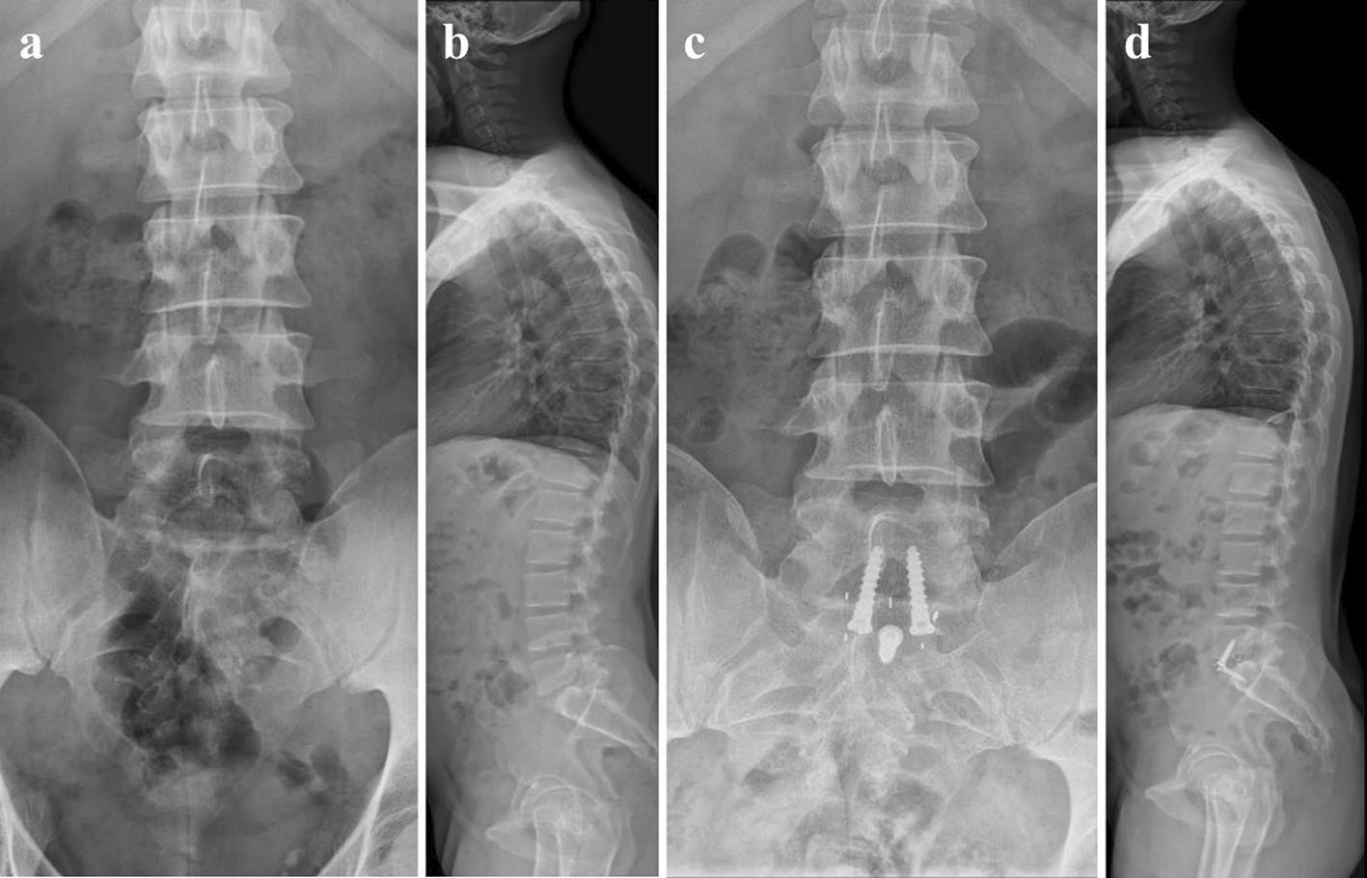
Case example. Pre- and postoperative radiographs of a 43-year-old patient with Castellvi grade Ib. (a,b) The patient’s preoperative radiographs while (c,d) depict radiographs taken after ALIF was performed.
